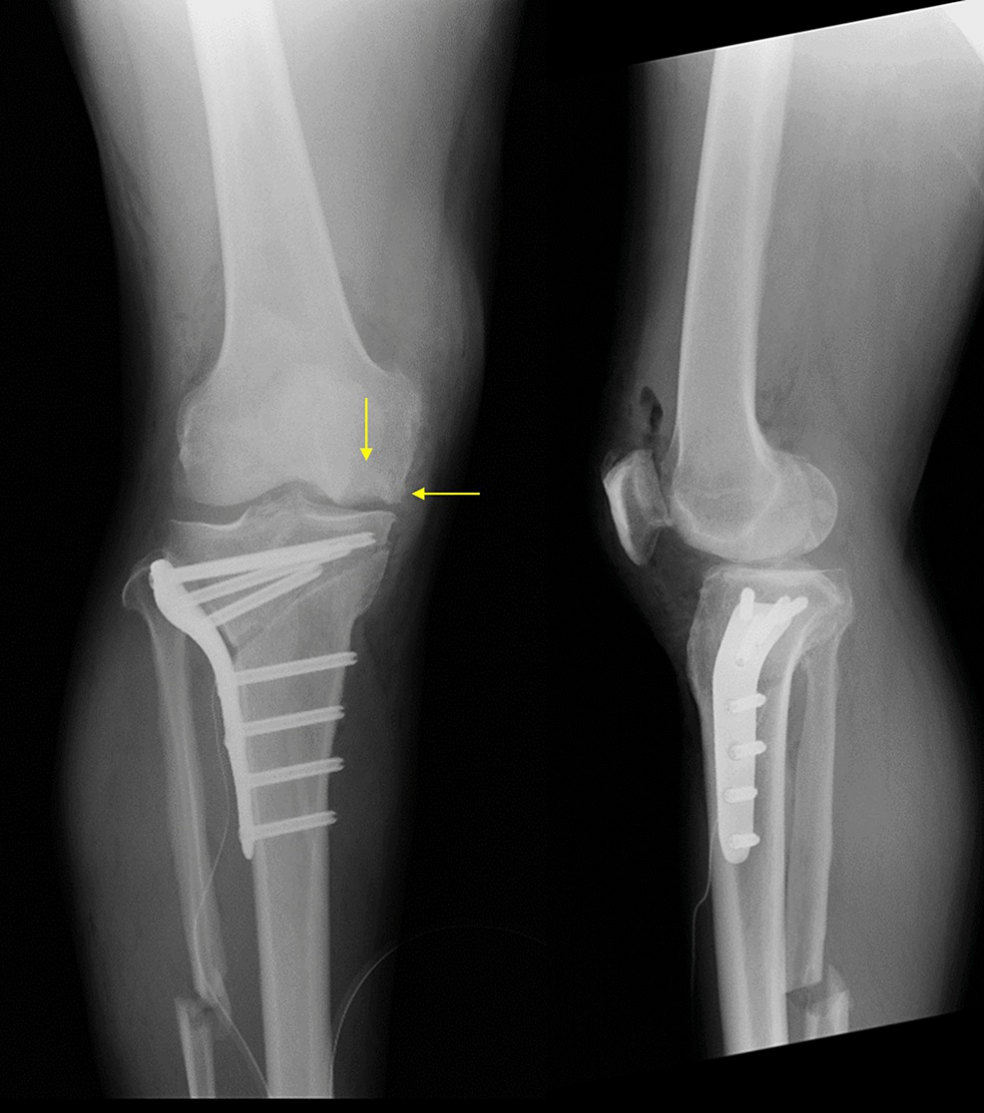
Postoperative radiograph of the right knee
